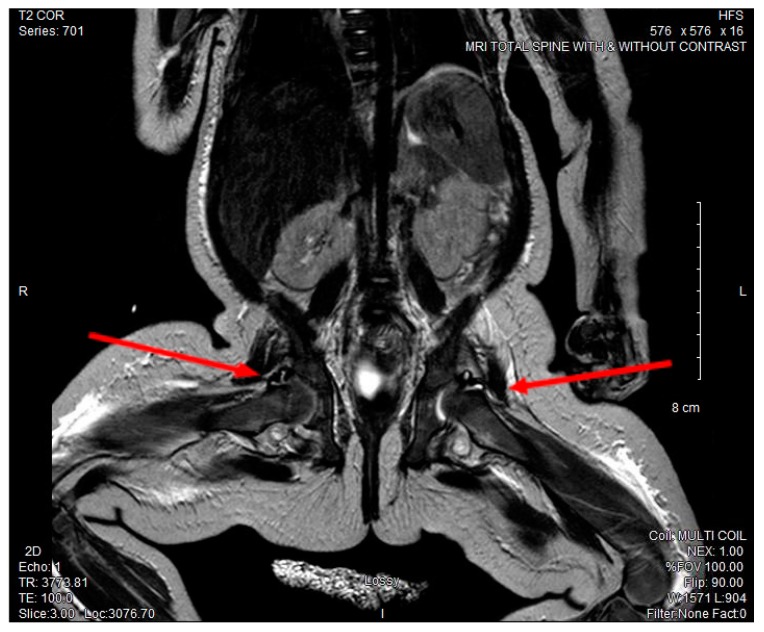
MRI full spine and hips with and without contrast. Abnormal T2 signal enhancement of bilateral hip joints.
